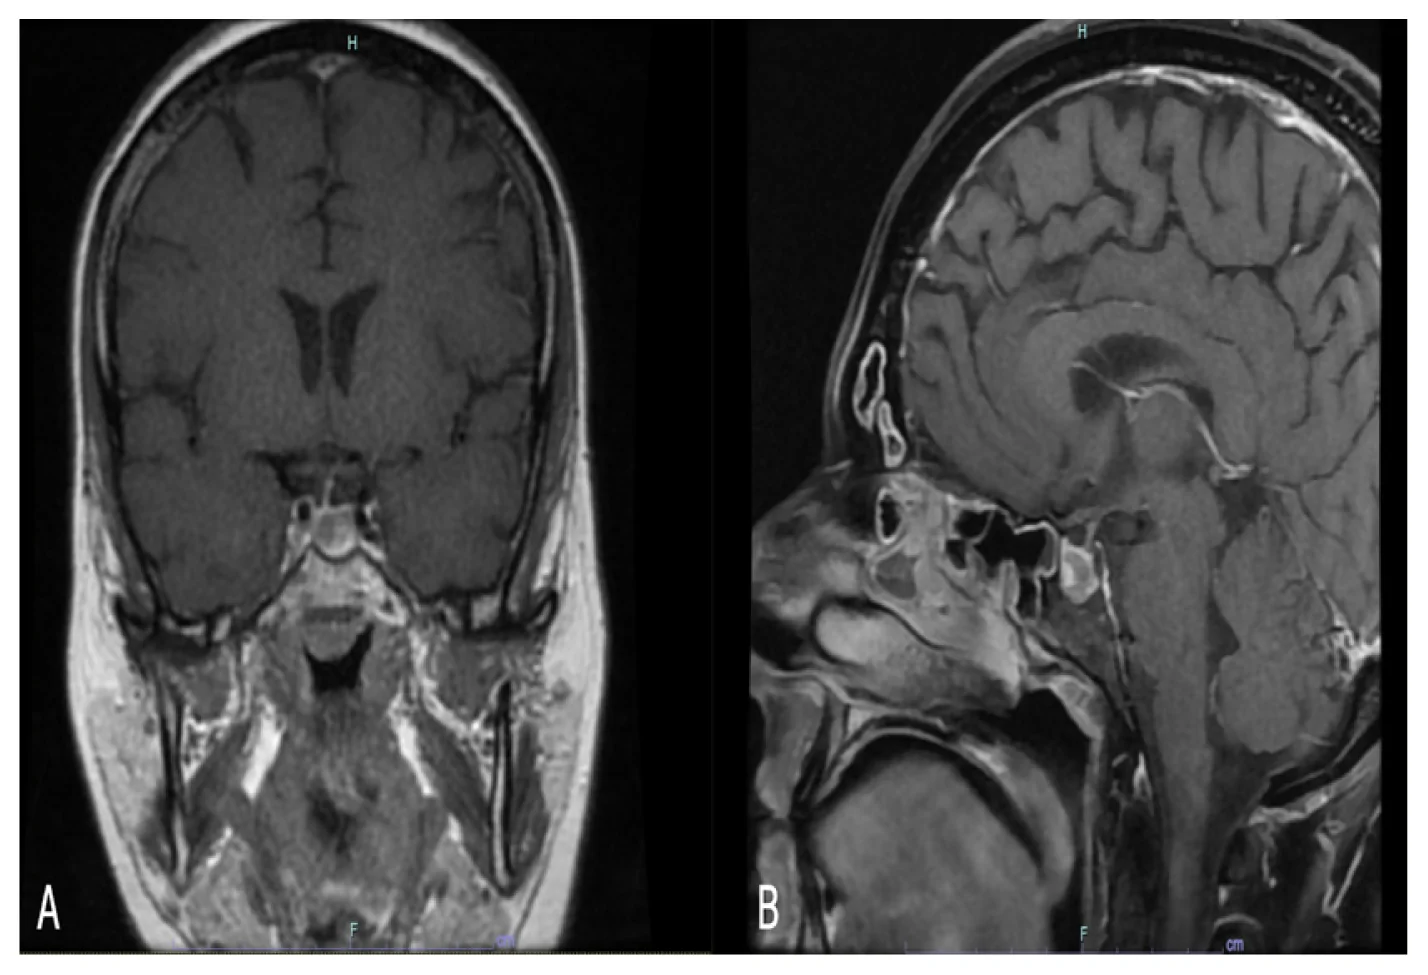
Pre-operative T1W magnetic resonance imaging scan with contrast in the (A) coronal and (B) sagittal view showing haemorrhagic pituitary microadenoma.
